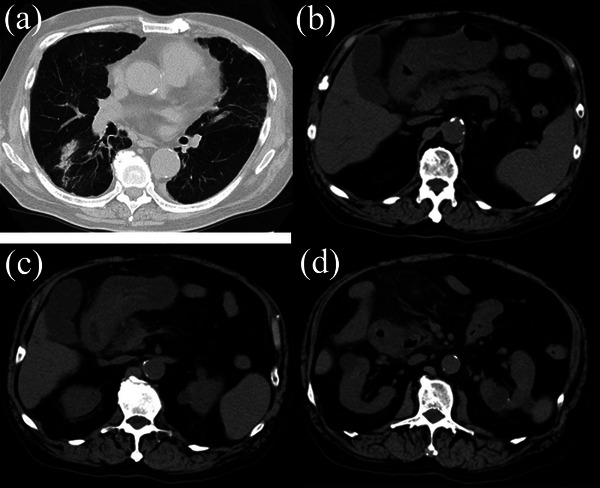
Computed tomography of the chest and abdomen. (a) Computed tomography of the chest reveals patchy opacification in the lower lobe of the right lung. This finding remains unchanged and is consistent with the previous diagnosis of cryptogenic organizing pneumonia. (b–d) Computed tomography of the abdomen shows significantly diffuse thickening of the gastric antrum and duodenum with fat stranding around these organs.
